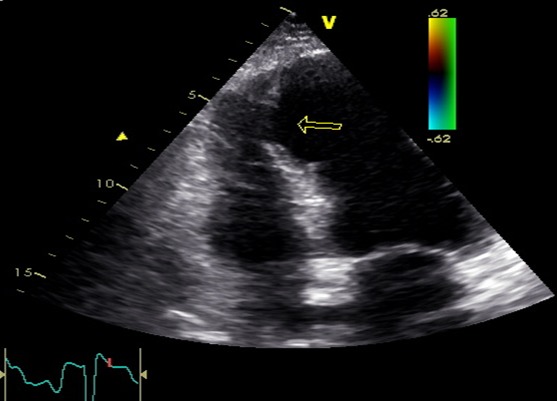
Aspect echocardiographique de la CIV
